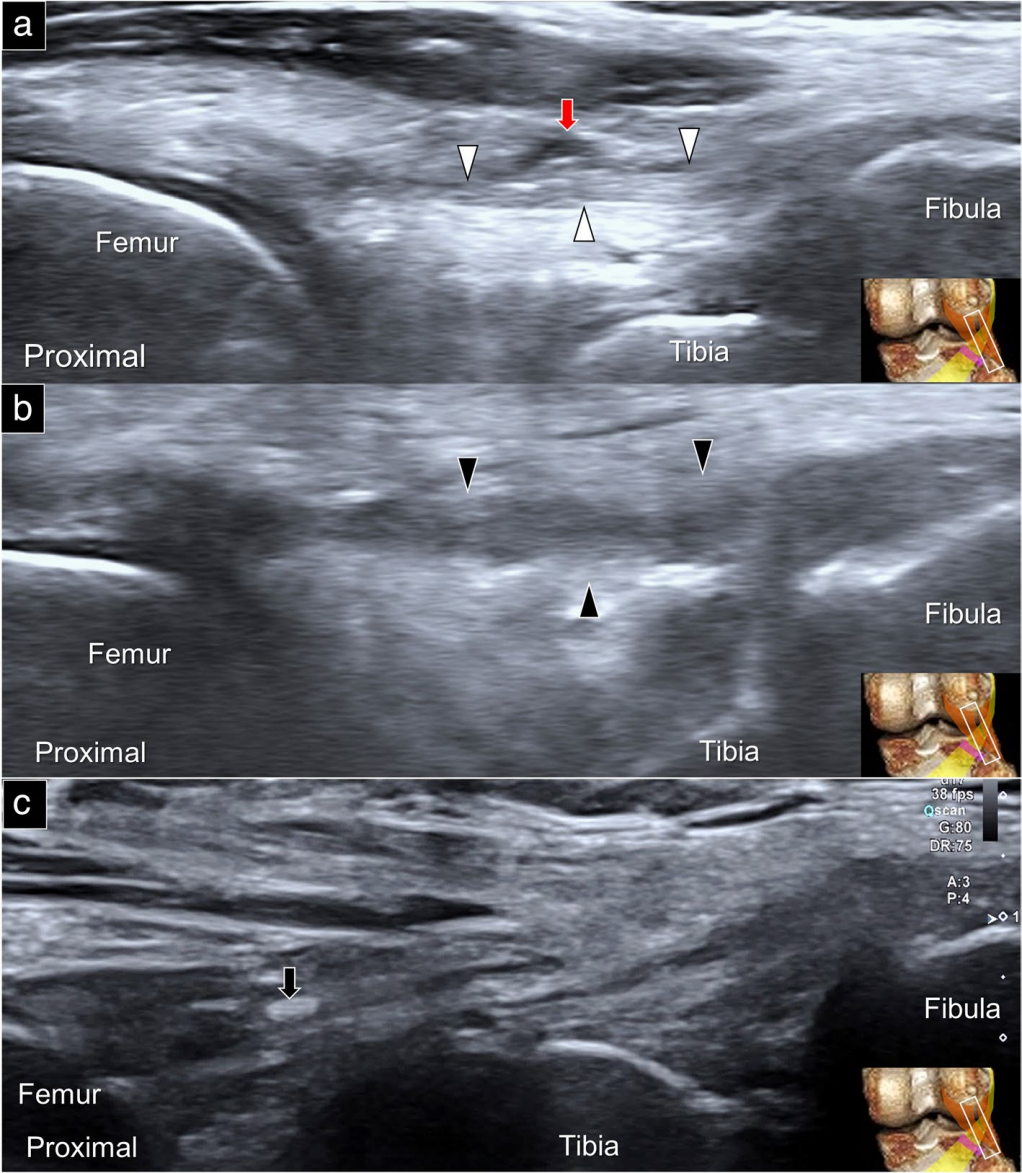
Sonographic imaging (long-axis view) depicts normal (white arrowheads) (a), thickened/hypoechoic (black arrowheads) (b), and calcified (black arrow) (c) arcuate ligaments. Note that the inferior lateral genicular artery (red arrow) is visible adjacent to the arcuate ligament
